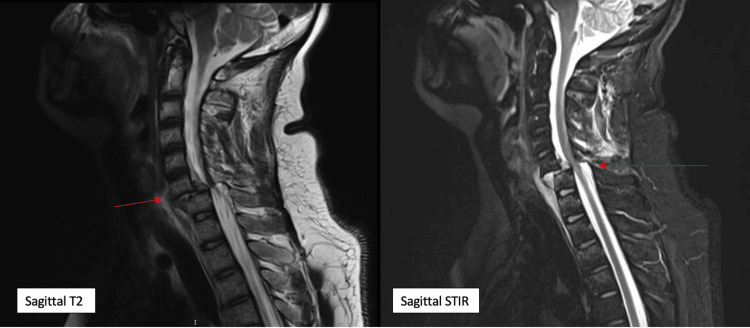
MRI sagittal T2 and STIR views showing C6-C7 fracture dislocation STIR: Short Tau Inversion Recovery
